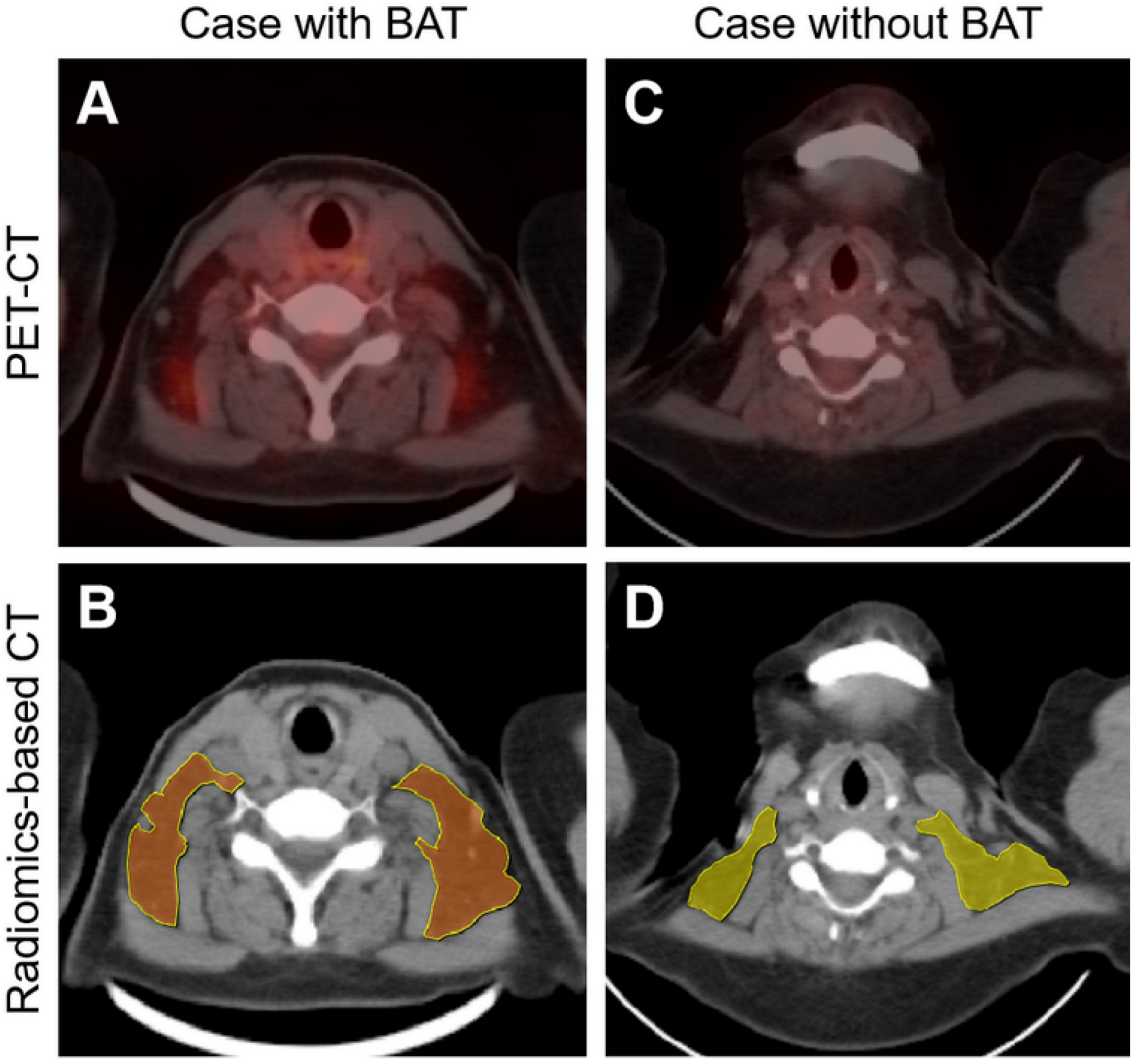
Radiomics-based brown adipose tissue detection on CT. PET-CT imaging (A and C) and radiomics-based CT imaging (B and D) of patients with or without brown adipose tissue (BAT). In the radiomics-based BAT detection model, BAT-positive and BAT-negative droplets are presented in brown (B) and yellow (D), respectively. Figure 5 is reproduced with permission from Ivyspring International Publisher (Theranostics. 2023; 13: 1584-93), an open access article under a CC-BY 4.0 license. BAT: brown adipose tissue; CT: computed tomography; PET: positron emission tomography.
